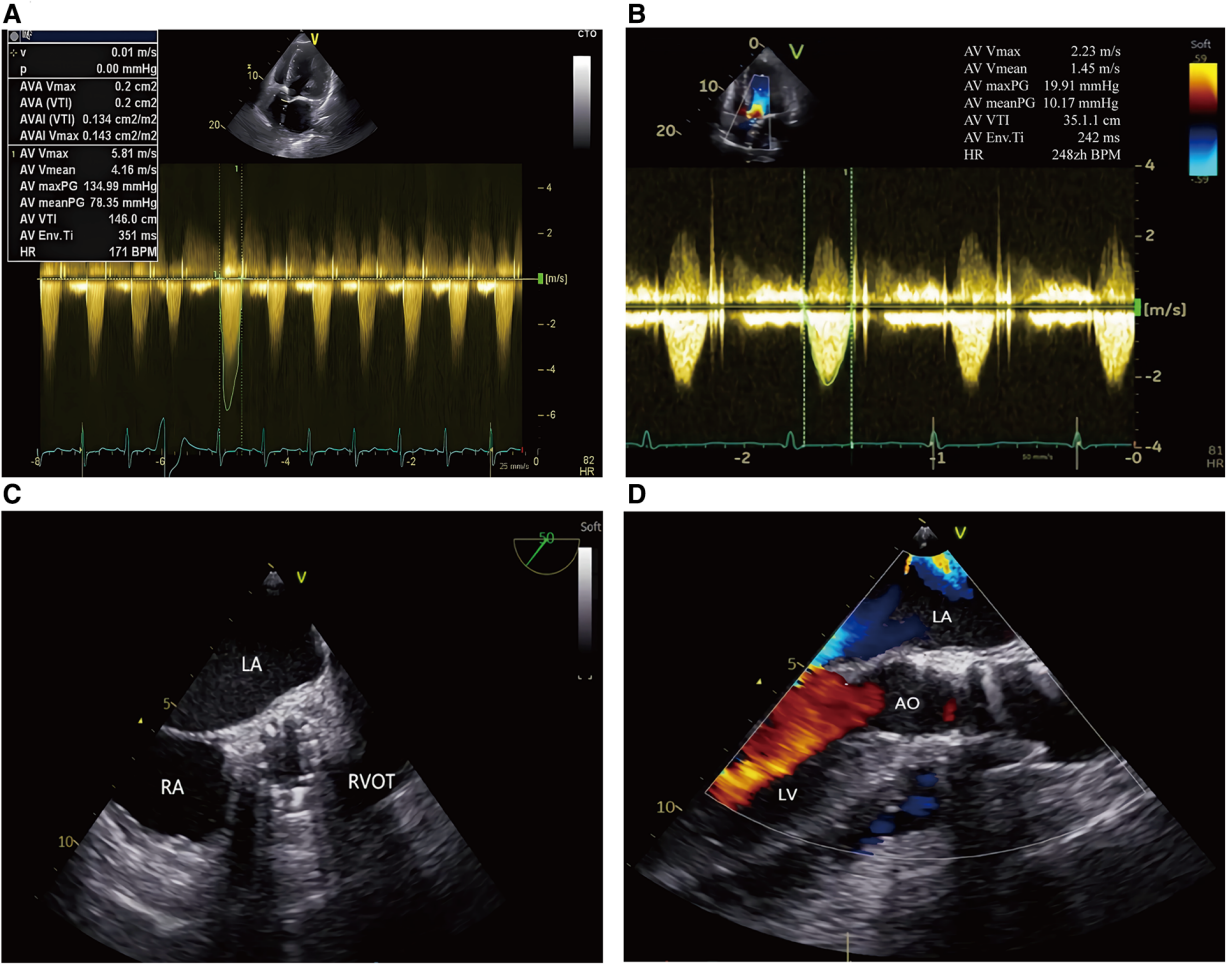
Trans-esophageal echocardiography in TAVR procedure. (A, B) Continuous Doppler showing the peak transvalvular pressure gradient: pre and post TAVR; (C) Postoperative echocardiography showed the prosthetic aortic valve with good morphology; (D) Postoperative echocardiography showed no significant aortic regurgitation after TAVR. LA, left atrium; RA, right atrium; LV, left ventricle; RVOT, right ventricular outflow tract; AO, aorta.
